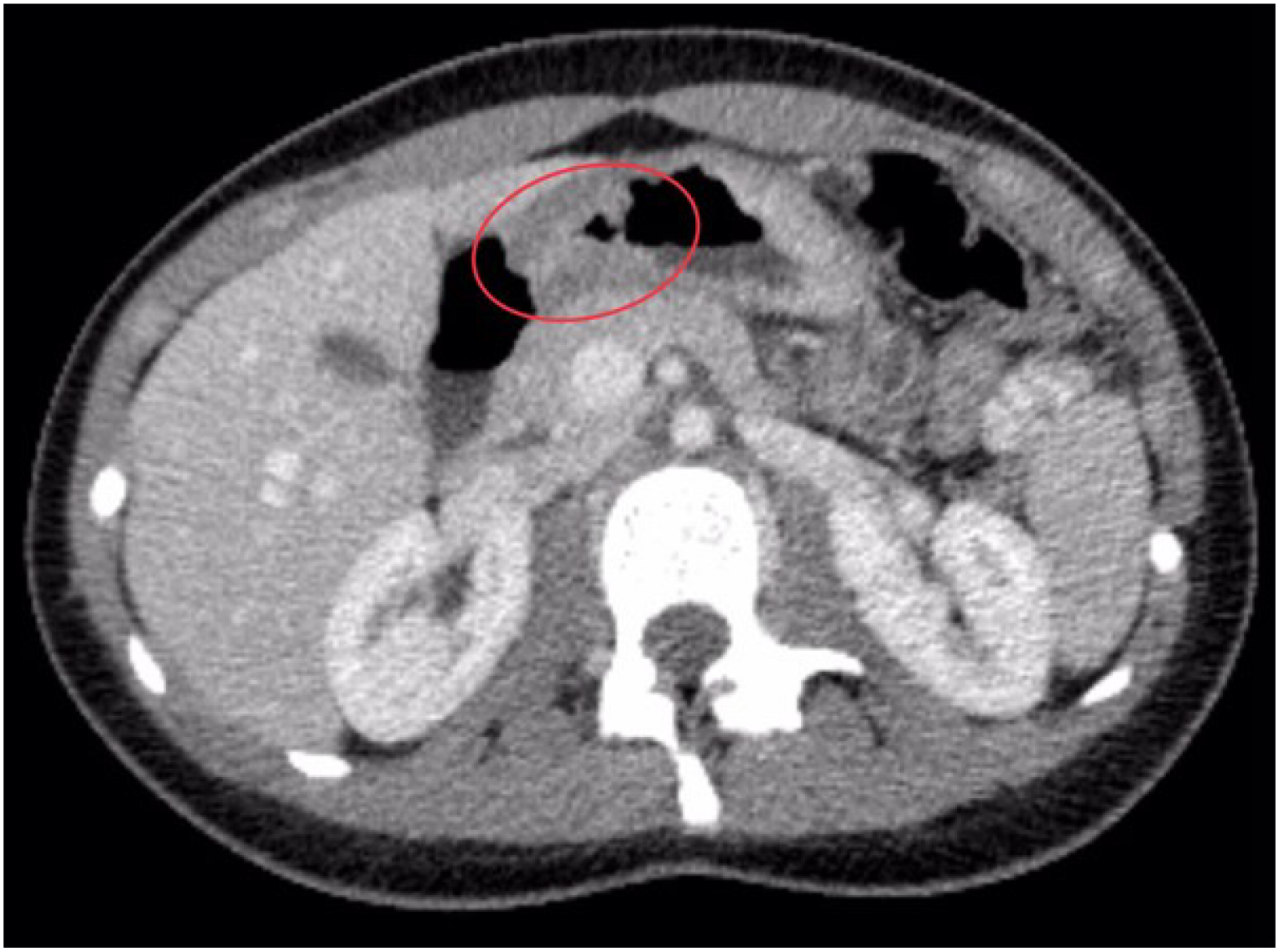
Axial contrast-enhanced CT image (portal venous phase) showing circumferential wall thickening of the gastric antrum extending into the pyloric region. The gastric wall demonstrates uniform thickening measuring approximately 9 mm (see red oval), associated with luminal narrowing and reduced contrast enhancement (iodophilic pattern). No signs of perigastric fat stranding or adjacent organ invasion are observed at this level. The radiological appearance raised strong suspicion for a primary gastric malignancy.
